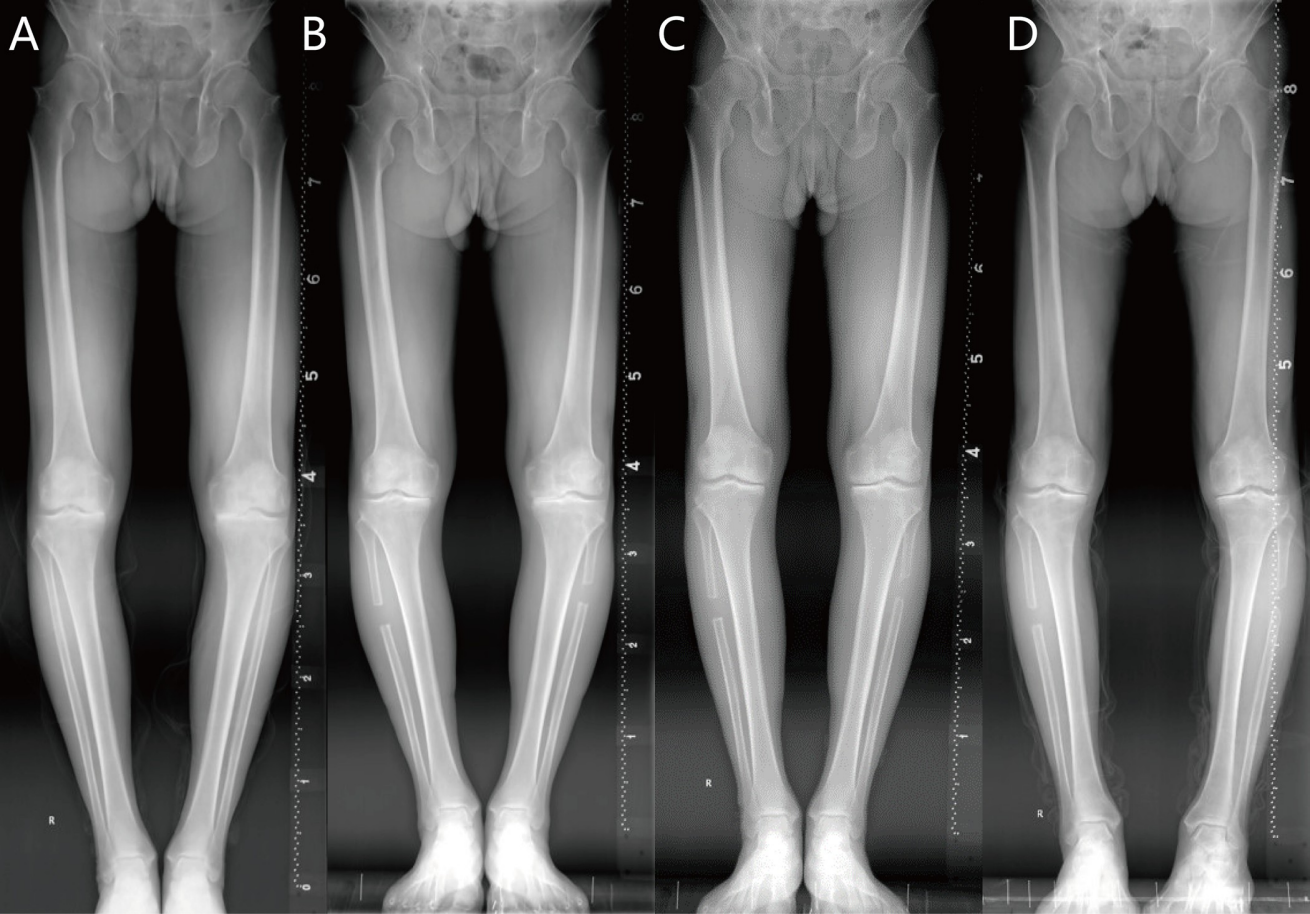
Preoperative and postoperative radiographs of a 68-year-old man. (A) Preoperative radiographs exhibited that a severe degree of medial compartment osteoarthritis. (B) Postoperative radiographs after PFO immediately, the alignment of knee and ankle was not changed obviously (C) Postoperative radiographs at 3 months after PFO shows that the recovered medial joint space and improved alignment of ankle (D) Postoperative radiographs at 1 years after PFO shows that medial joint space and improved alignment of ankle was maintained with some decrease.
